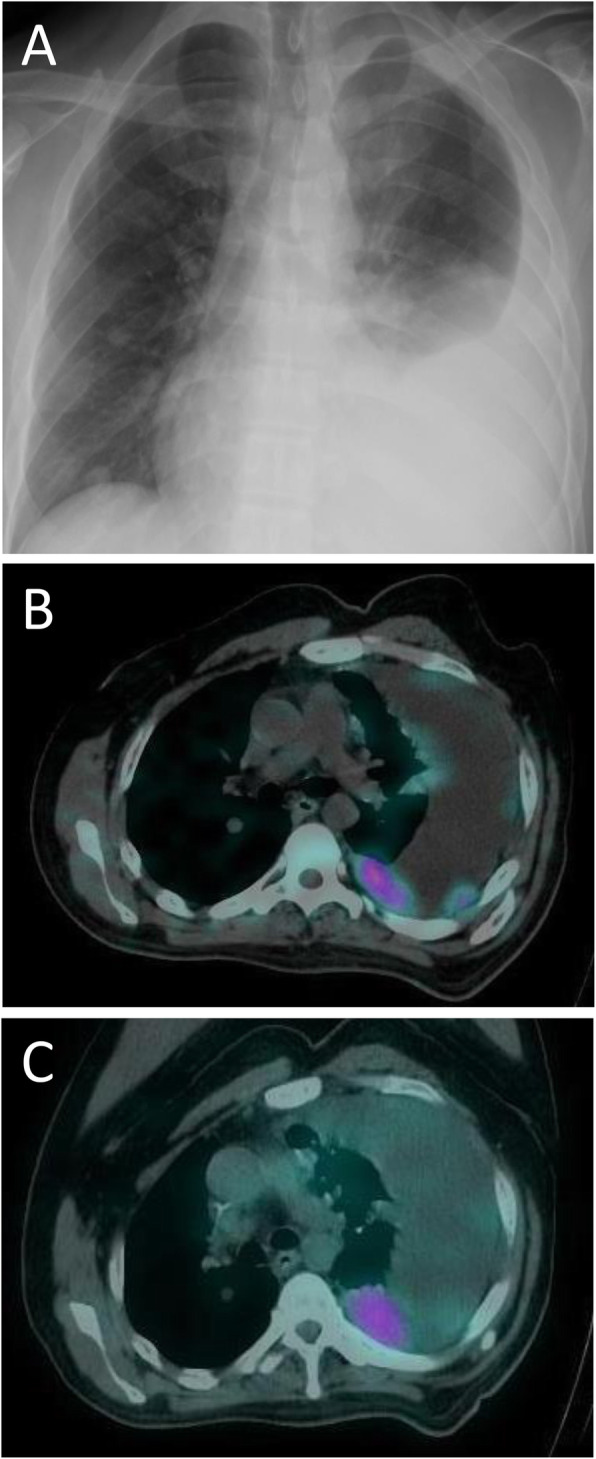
Preoperative imaging findings. a Chest X-ray showing massive pleural effusion. b MIBI scintigraphy and c somatostatin receptor scintigraphy showing functionally active status in left pleural dissemination, but not in the area of pulmonary metastasis
